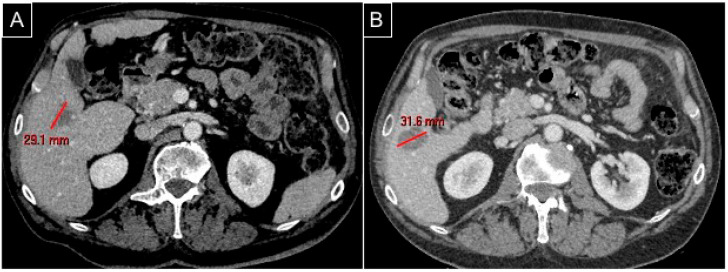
The same patient as Figure 1. (A) A preprocedural CT scan in the venous phase axial plane detecting the hypovascularized metastasis in segment V; (B) a postprocedural CT scan in the venous phase axial plane for follow-up at 2 months showing complete ablation with an ovoid regular margin without remnant.
